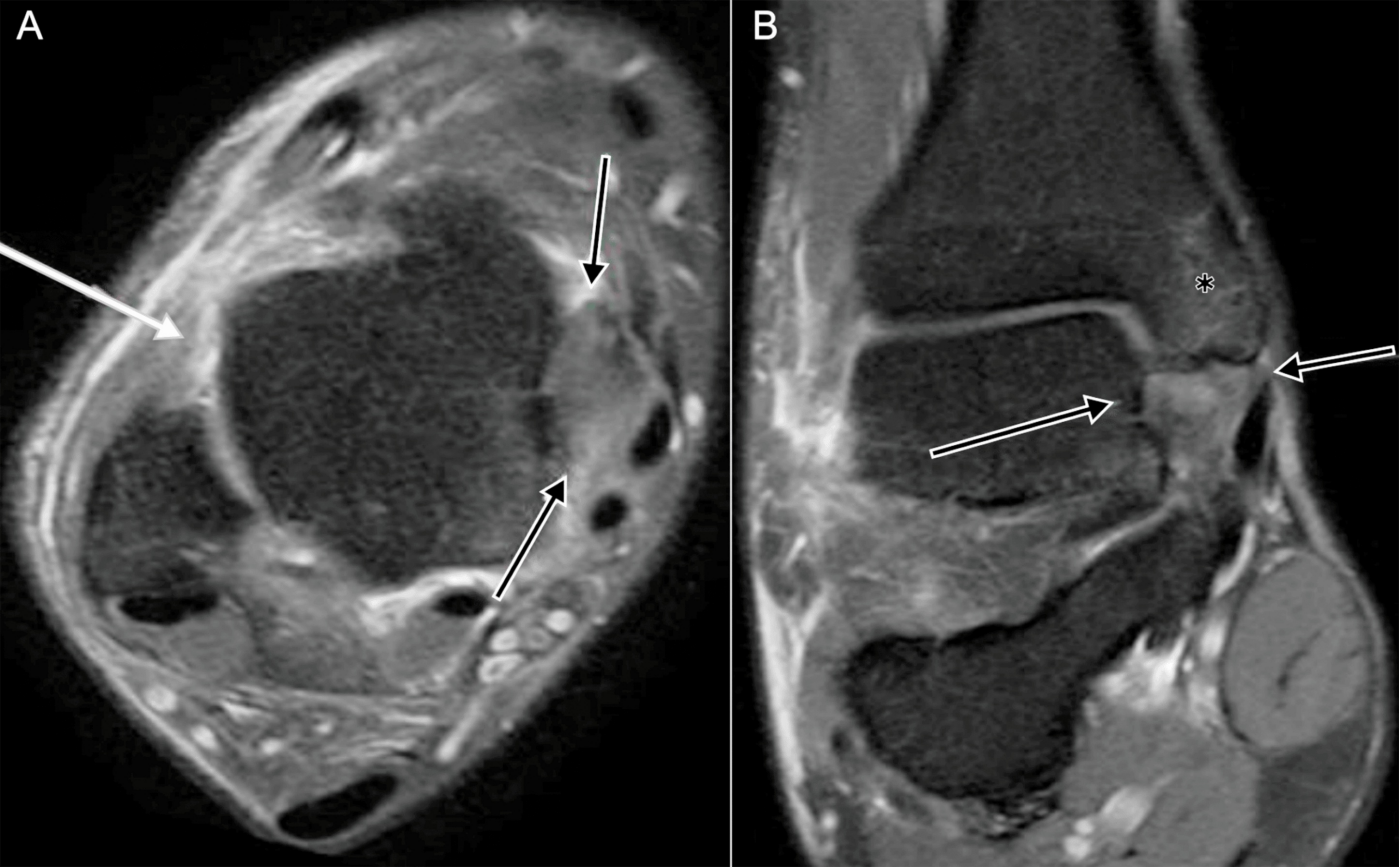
Anterolateral ligament and deltoid complex injury on MRI. A 16-year-old male: (A) axial DPFS MRI shows discontinuity of the anterolateral ligament (white arrow) and a partial tear of the deep deltoid fibers (black arrows); (B) coronal DPFS MRI demonstrates a deep deltoid fiber tear (black arrows) and tibial marrow edema (*). DPFS: dual proton fat-saturated sequence Images belong to the authors; patient consent was obtained.
